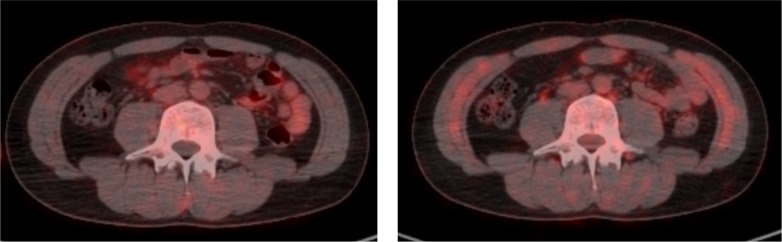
Representative positron emission tomography-computed tomography axial images of the trunk. (Left) the control study (Right) the exercise study.
